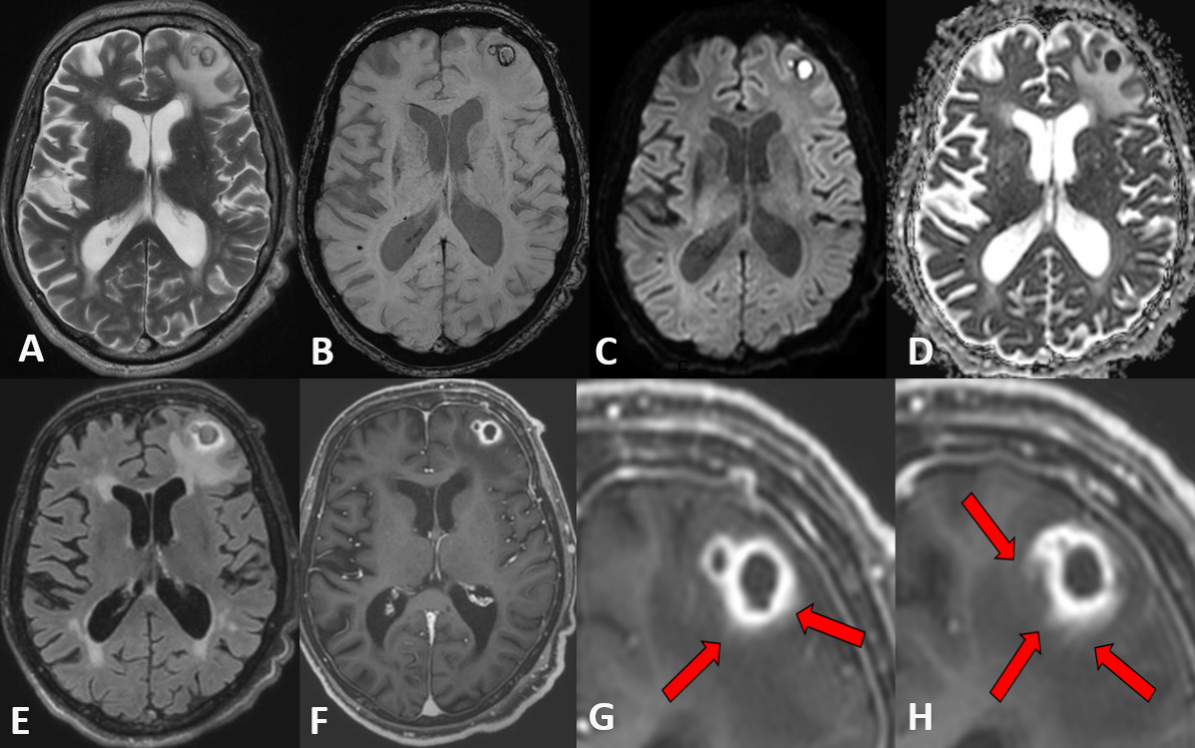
Transverse *T*_2_W TSE (A) , SWI (Figure 1B), DWI (Figure 1C), ADC map (Figure 1D) and FLAIR (Figure 1E) images and post-contrast *T*_1_W TFE (Figure 1F–1H) presenting the two peripherally contrast enhancing confluent lesions in the left frontal white matter Red arrows show indistinct contrast enhancement in the surrounding tissue. ADC, apparent diffusion co-efficient; DWI,diffusion-weighted imaging; FLAIR, fluid attenuated inversion recovery; SWI, susceptibility-weighted imaging; *T*_2_W, *T*_2_weighted; TFE, turbo field echo.
